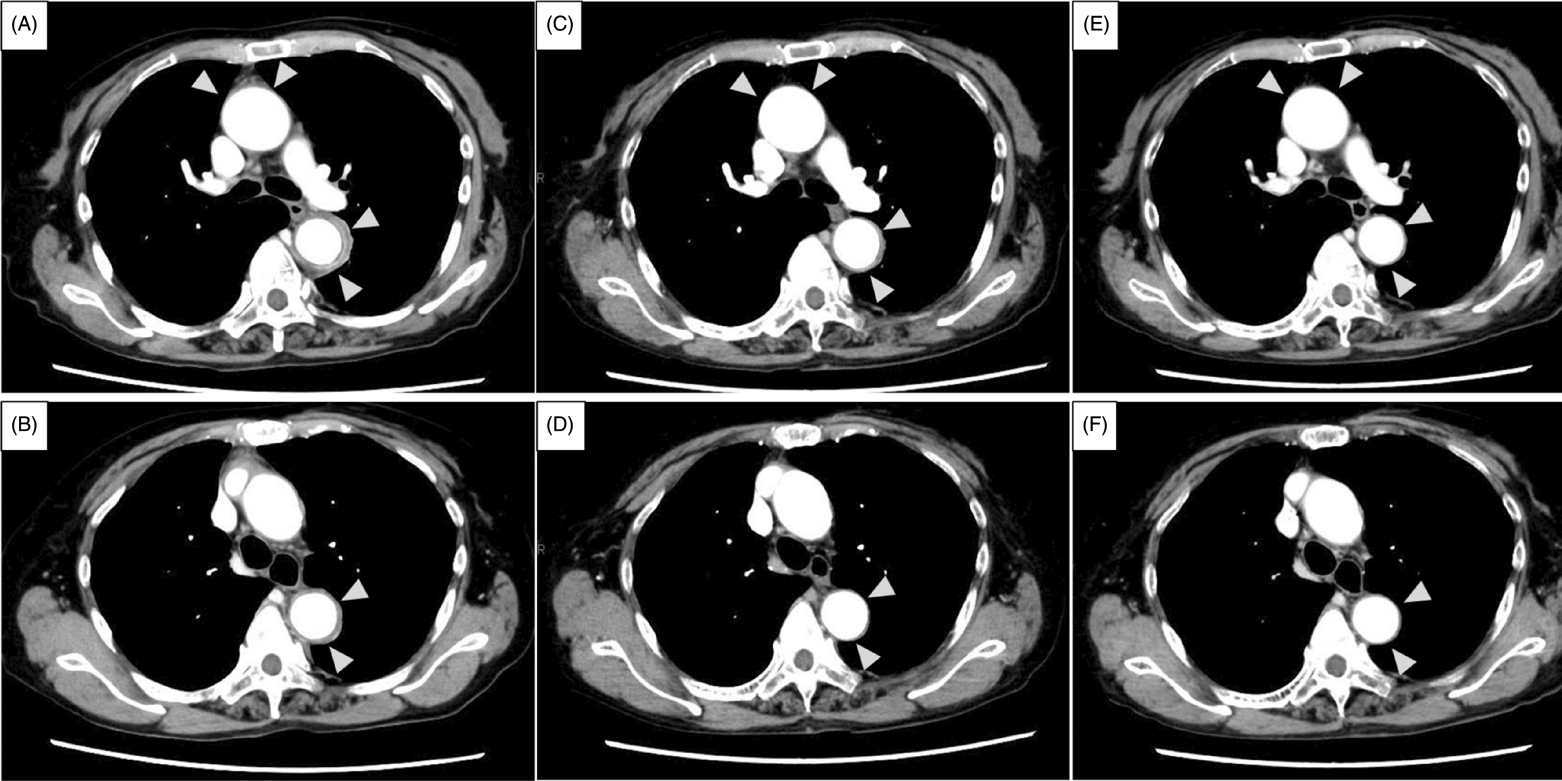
(A, B) Contrast‐enhanced computed tomography (CE‐CT) on day 15 after the first pegfilgrastim administration showing soft tissue thickening around the descending aorta and brachiocephalic artery (white arrowheads). (C, D) CE‐CT findings on day 22 after the first pegfilgrastim administration showing improvement. (E, F) CE‐CT findings on day 39 after the first pegfilgrastim administration showing complete disappearance.
